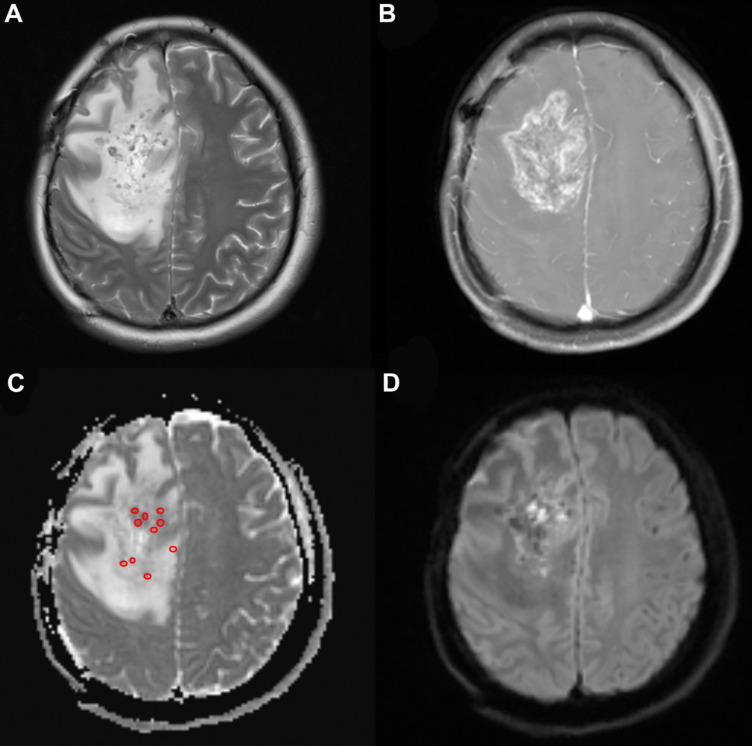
A 33-year-old woman with high-grade glioma (WHO grade IV). (a) Pre-^125^I implantation T2WI, (b) contrast-enhanced T1WI, (c) ADC map (circles indicate the ADC values measured on the ADC map), and (d) DWI showing the glioma location in the brain. The minimum ADC value was detected on the ADC map.
